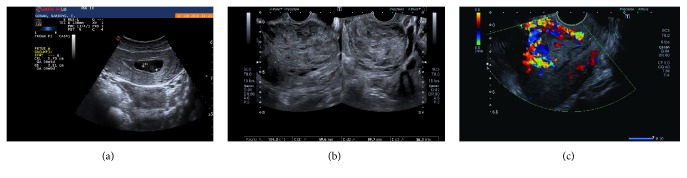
(a) Ultrasound image just before D/C shows 9-week fetus with no cardiac activity and there is no sign of uterine AVM. (b) Sagittal endovaginal image of the uterus shows 60 × 60 × 56 mm (103 cm^3^) hyperechogenic and heterogeneous mass lesion located in the anterior wall of the uterus and extending laterally at the left. (c) Color Doppler image shows multiple tortuous vessels.
